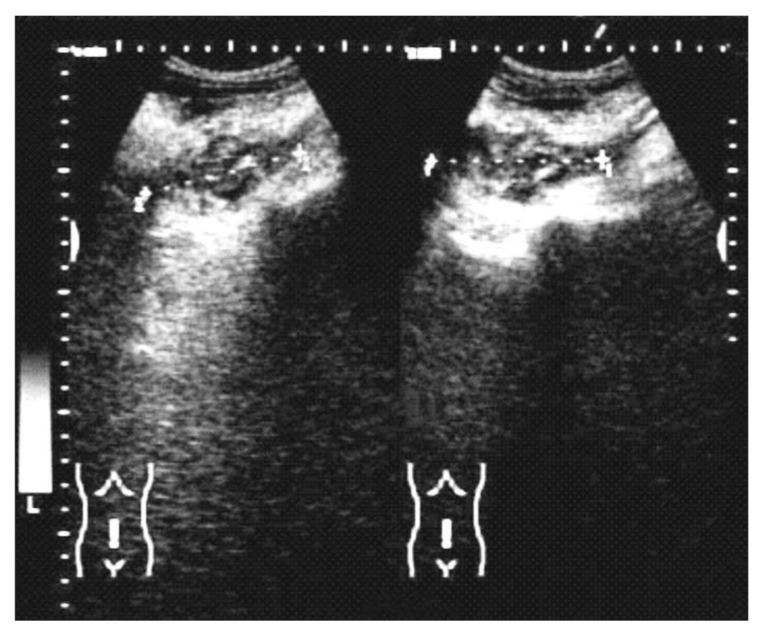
Abdominal ultrasound discovered a heterogeneous abdominal tumor and peritumoral fluid.
